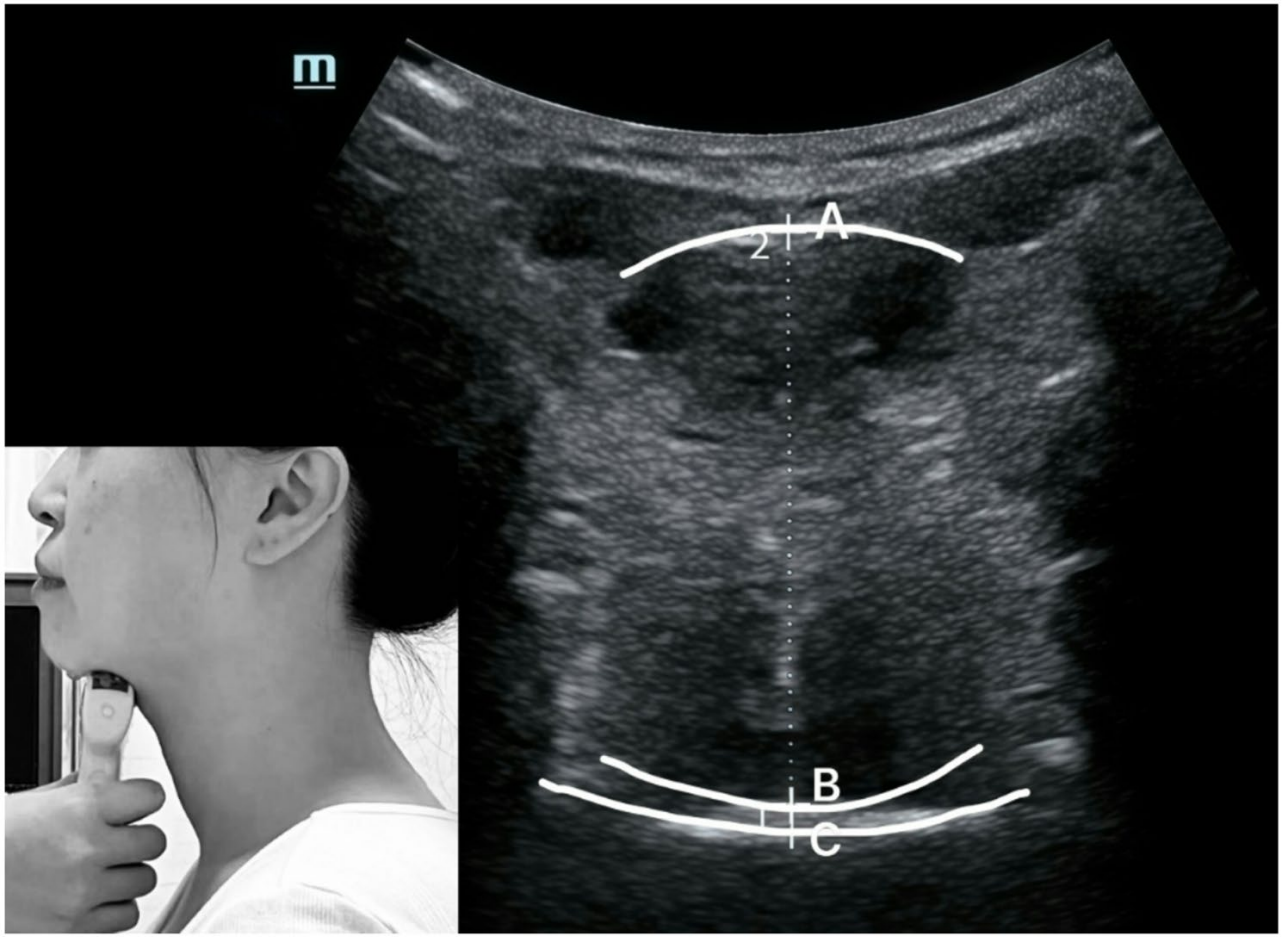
Ultrasound image scanned on the frontal plane of tongue. A: Basal lingual sole; B: Dorsal side of tongue; C: Surface of the palate. AB: Tongue thickness, BC: Tongue-palate distance
